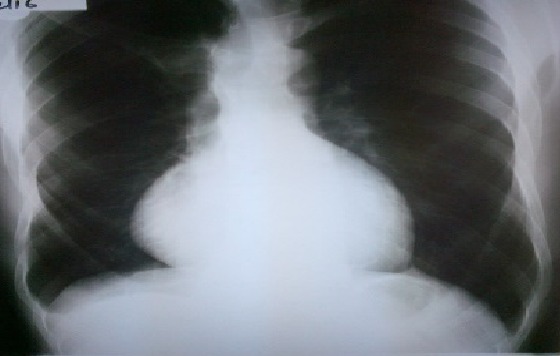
Chest radiograph of index case
